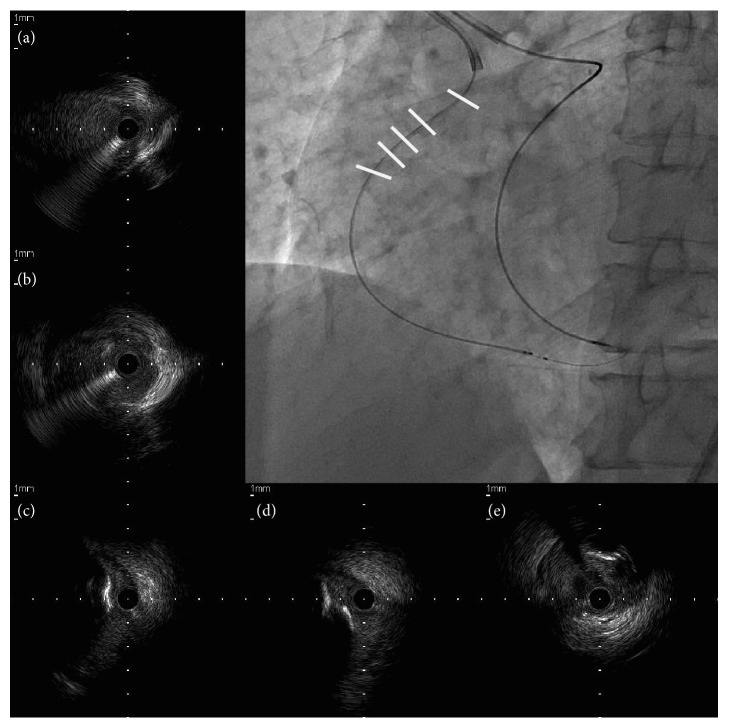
Intravascular ultrasound (IVUS) examination. IVUS was performed after guidewire externalization. Serial IVUS images of right coronary artery (RCA) show the ostial RCA (a), the guidewire entering into the true lumen from the false lumen at the proximal RCA (b), and the guidewire passing the chronic total occlusion (CTO) segment through the subintimal space, avoiding the severely calcified plaque at the proximal segment of CTO ((c)–(e)). Images are viewed in a proximal (a) to distal (e) direction.
